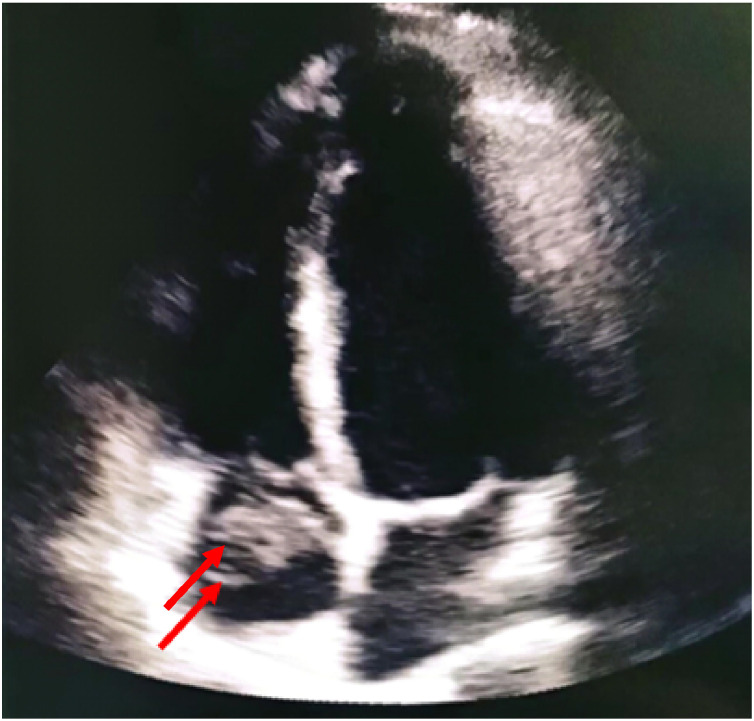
Apical 4-chamber transthoracic echocardiography showing a right atrial thrombus (red arrow).
